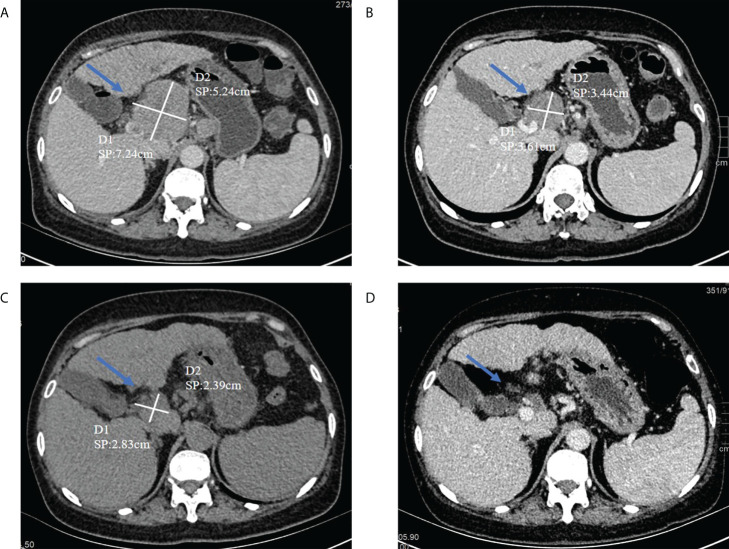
(A) On August 6, 2021, abdominal CT (before treatment) had a tumor in the hepatic hilum area, with a size of about 5.24*7.64cm (indicated by the arrow); (B) The tumor in the hepatic hilum area on September 25, 2021, about 3.44*3.61cm in size (indicated by the arrow); (C) On January 20, 2022, abdominal CT (after treatment) had a tumor in the hepatic hilum area, with a size of about 2.39*2.83cm(indicated by the arrow); (D) On Apirl 23, abdominal CT showed that the tumor had basically disappeare.
